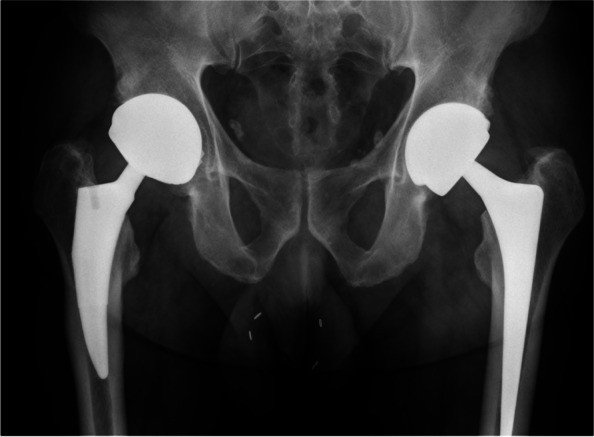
Postoperative anteroposterior radiograph after implantation of the left THA
